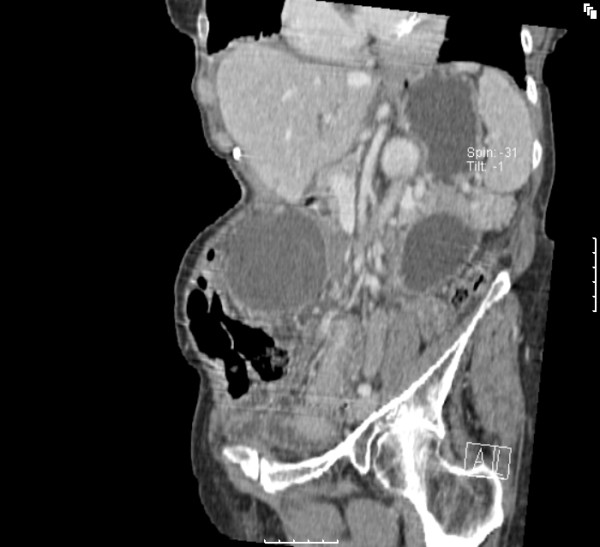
CT scan of the abdomen and pelvis in sagittal section.
